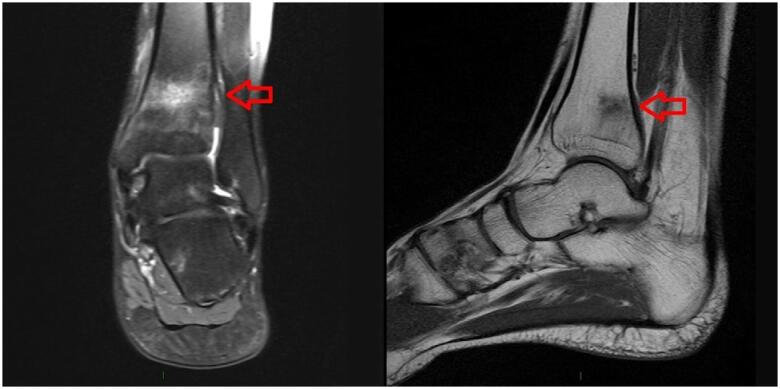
Magnetic resonance imaging showing coronal T2 and sagittal T1 views of the patient's fracture in the left distal tibia.
